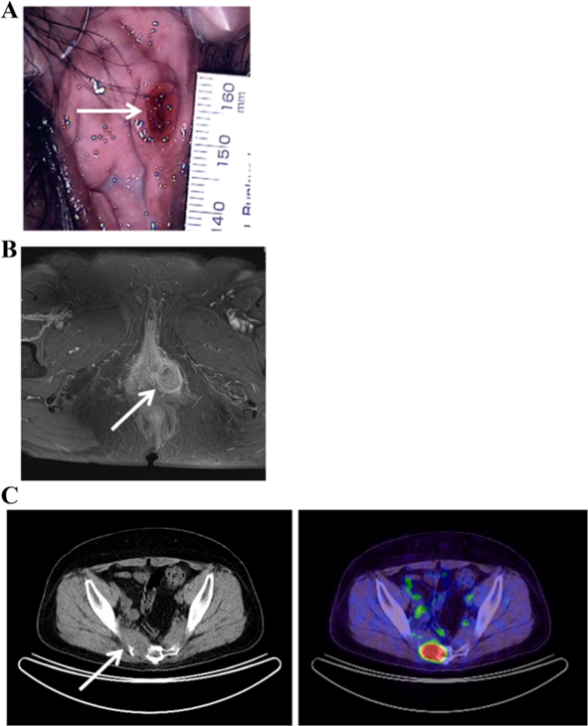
Physical examination and clinical imaging. a Ulcerative lesion on the inner side of the left labium minorum (*arrow*). b T1-weighted, contrast-enhanced magnetic resonance image revealing a 31 × 24 mm mass of moderate-to-high intensity in the left vulvar region (*arrow*); the mass does not involve the urethra or anus. c Positron emission tomography computed tomography revealing tumor metastases to the sacrum (*arrow*)
